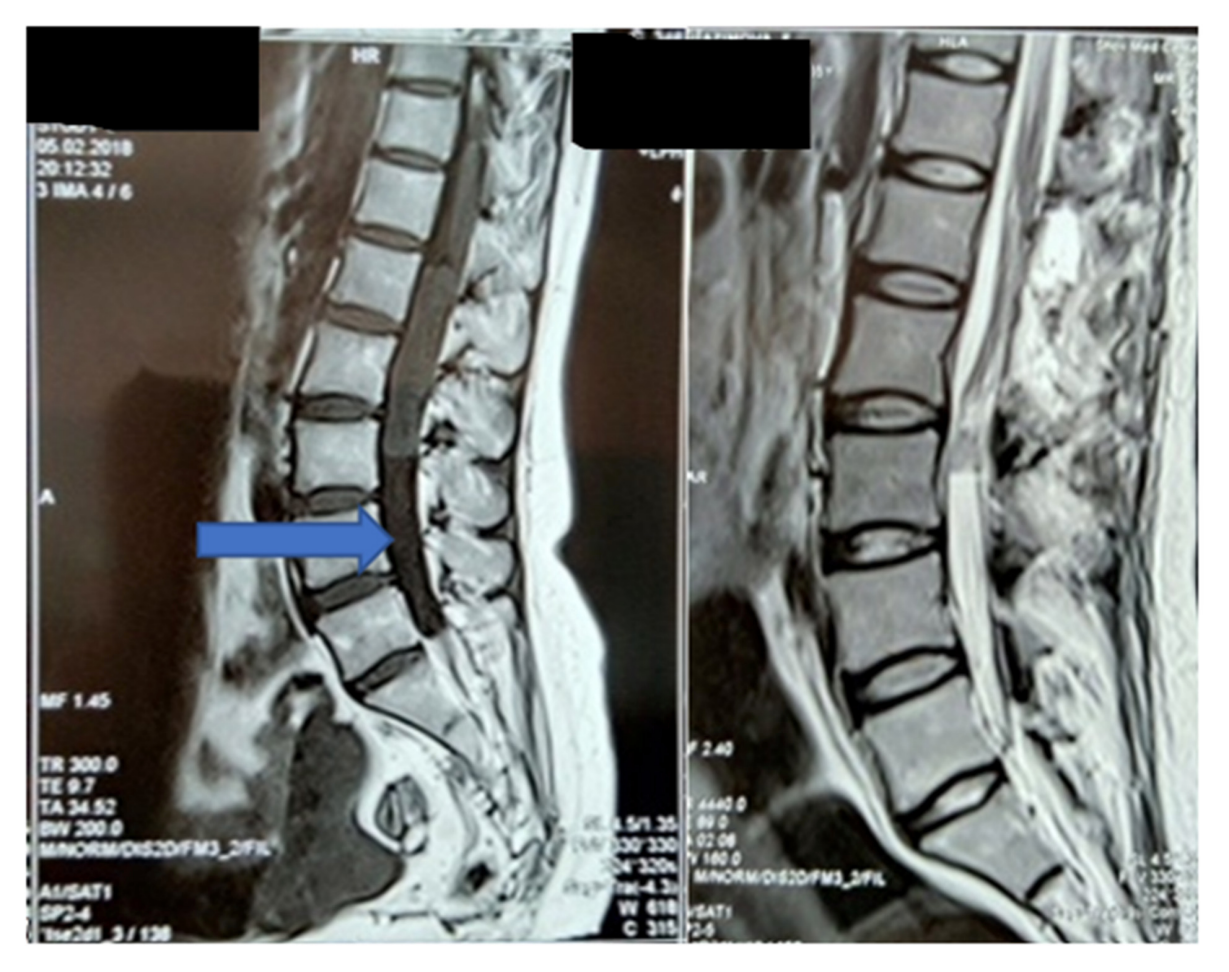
MRI of the cervical spine, preoperative and 2 days post-surgery. A 36-year-old with a Neuroma on the ventral surface of the spinal cord, at the level of C1-C2 (blue arrow). Control MRI shows total tumor removal using an improved method (orange arrow).
